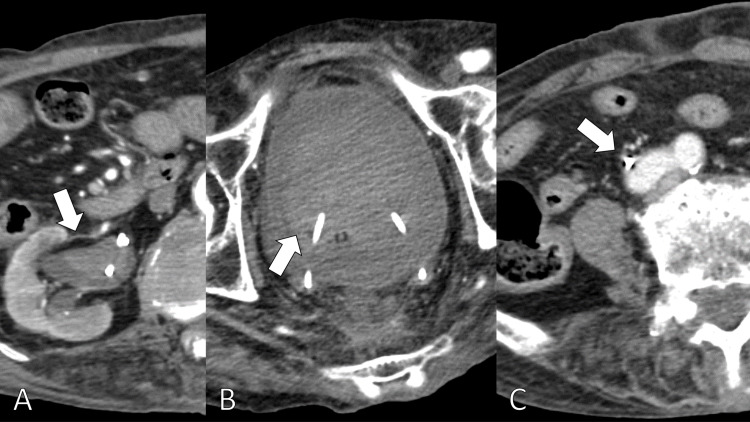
Contrast-enhanced CT revealing the higher density area in the right renal pelvis (A), higher density areas in the urinary bladder (B), and the right common iliac arterio-ureteral fistula (C).
